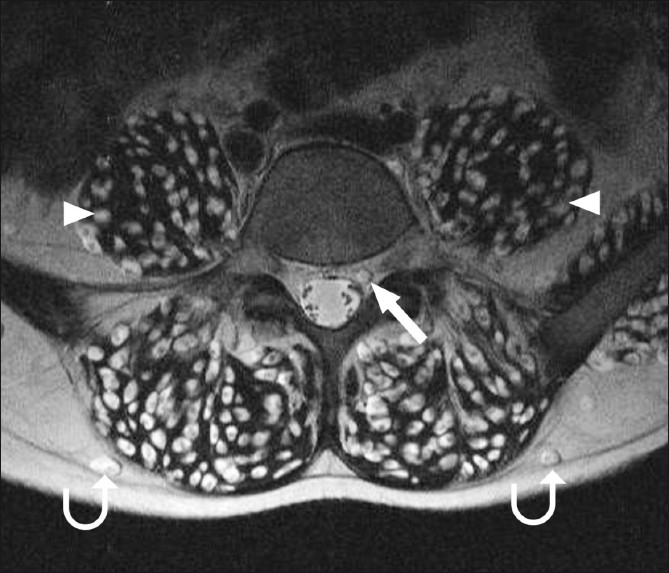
T2W axial MRI of the lumbar spine shows cysticercus cysts in the extradural spinal space (arrow), in addition to muscle (arrowhead) and subcutaneous (curved arrow) involvement
